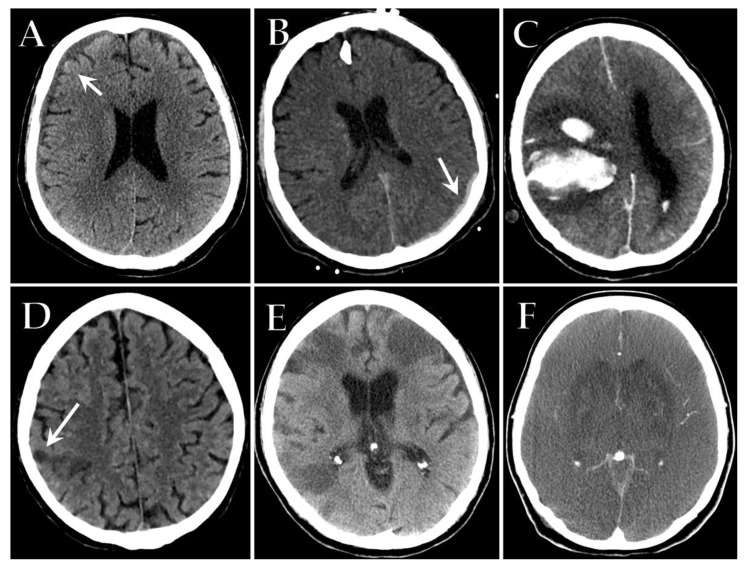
Axial unenhanced CT images of different patients with severe COVID-19 admitted to ICU showing spontaneous subacute right frontoparietal subdural hematoma (white arrow) (A), acute left parieto-occipital subdural hematoma (white arrow) (B) and massive right intracerebral hemorrhage with surrounding edema and significant midline shift (C). Axial unenhanced CT images show an acute ischemic stroke in the right parietal lobe (white arrow) (D) and multiple bilateral acute ischemic strokes (E) in two different COVID-19 patients admitted to ICU. Axial contrast-enhanced CT of a COVID-19 comatose patient reveals diffuse sulcal effacement with a decreased differentiation between gray and white matter, compatible with severe anoxic brain injury (F).
